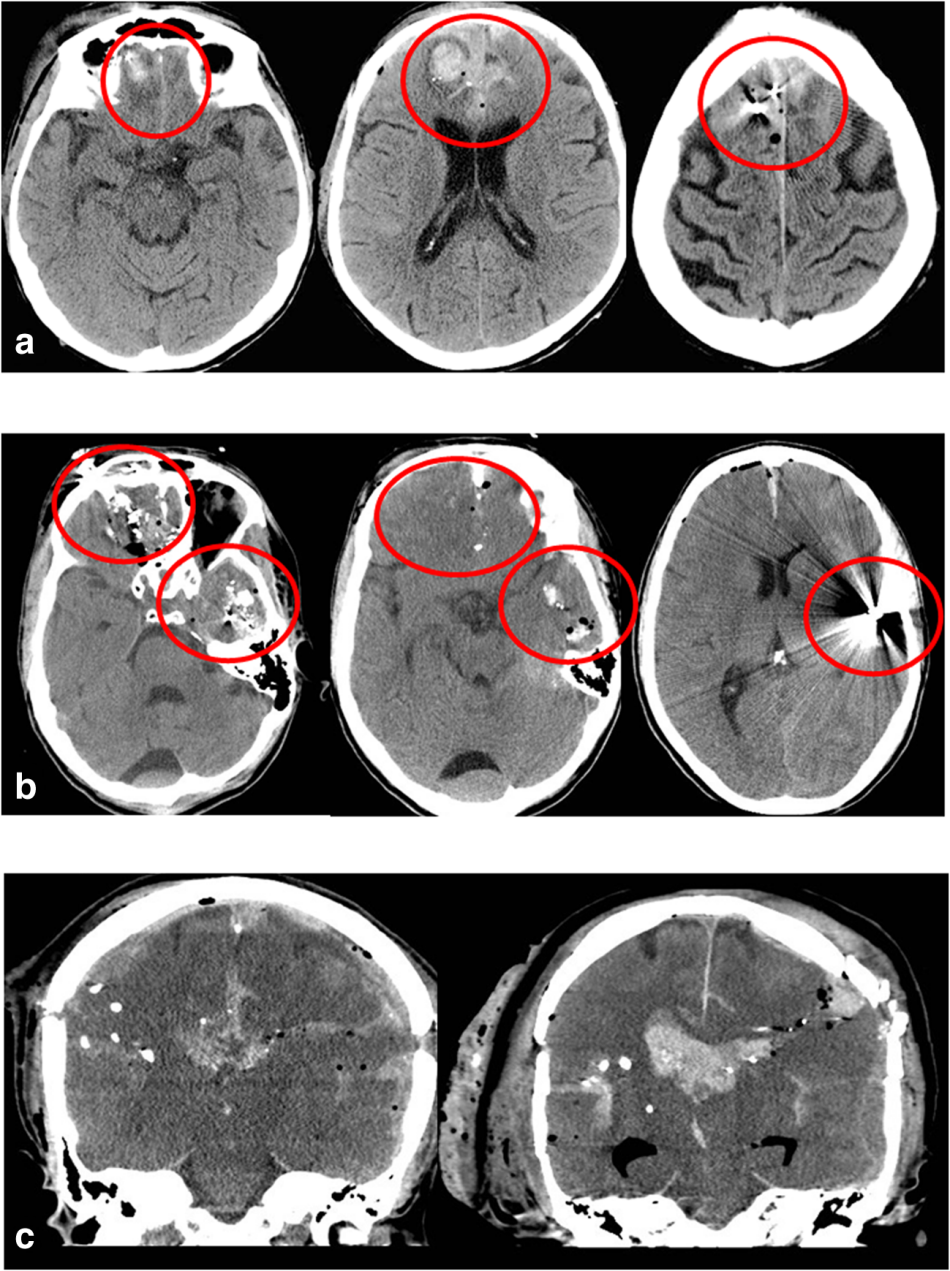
Examples of head CT scans in patients with gunshot wound with brain injury (GSWB). Patient A suffered injury of both frontal lobes without CT scan visible damage to the basal ganglia, the thalamus, or the ventricles. The three serial axial sections demonstrate the bullet path through the frontal lobes (shot through the palate, bullet remaining intracranially in the right superior frontal gyrus). Patient B demonstrates another example of GSWB causing damage to multiple lobes (both frontal lobes and the left temporal lobe) without affecting the basal ganglia, thalamus, or the ventricles. The injuries of Patient A and B are survivable despite extensive damage. The coronal CT scan sections in C demonstrate examples of the bullet tract passing through the ventricles in two different patients. This kind of gunshot injury was clearly associated with poor prognosis and can be deemed unsurvivable in most cases
